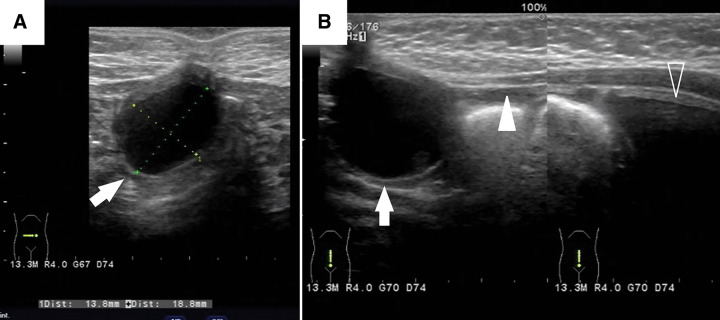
Ultrasound findings at 4 months of age. (A) A well-defined cyst measuring 2 cm in diameter is identified beneath the umbilicus (arrow). (B) A linear echogenic structure (solid arrowhead) extending from the cyst (arrow) to the urinary bladder (open arrowhead) is noted on sagittal scanning, suggesting a persistent urachal remnant.
